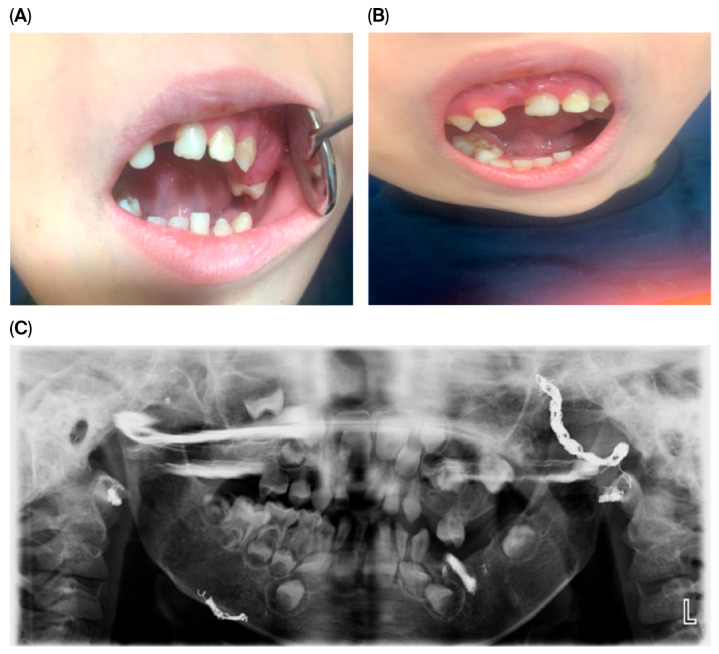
Intraoral and extraoral clinical images at the initial examination (age 4). (A) Intraoral view showing gingival overgrowth in the upper molar region. (B) Extraoral frontal view demonstrating facial asymmetry and prominent cheeks. (C) Panoramic radiograph showing multilocular radiolucent lesions within alveolar bone.
